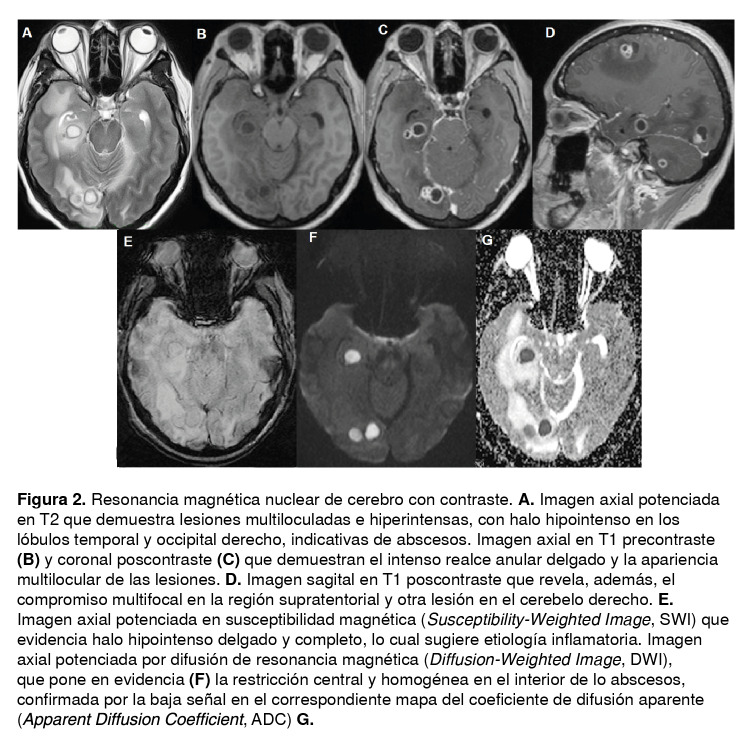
Resonancia magnética nuclear de cerebro con contraste. A. Imagen axial potenciada en T2 que demuestra lesiones multiloculadas e hiperintensas, con halo hipointenso en los lóbulos temporal y occipital derecho, indicativas de abscesos. Imagen axial en T1 precontraste (B) y coronal poscontraste (C) que demuestran el intenso realce anular delgado y la apariencia multilocular de las lesiones. D. Imagen sagital en T1 poscontraste que revela, además, el compromiso multifocal en la región supratentorial y otra lesión en el cerebelo derecho. E. Imagen axial potenciada en susceptibilidad magnética *(Susceptibility-Weighted Image,* SWI) que evidencia halo hipointenso delgado y completo, lo cual sugiere etiología inflamatoria. Imagen axial potenciada por difusión de resonancia magnética *(Diffusion-Weighted Image,* DWI), que pone en evidencia (F) la restricción central y homogénea en el interior de lo abscesos, confirmada por la baja señal en el correspondiente mapa del coeficiente de difusión aparente *(Apparent Diffusion Coefficient,* ADC) G.
